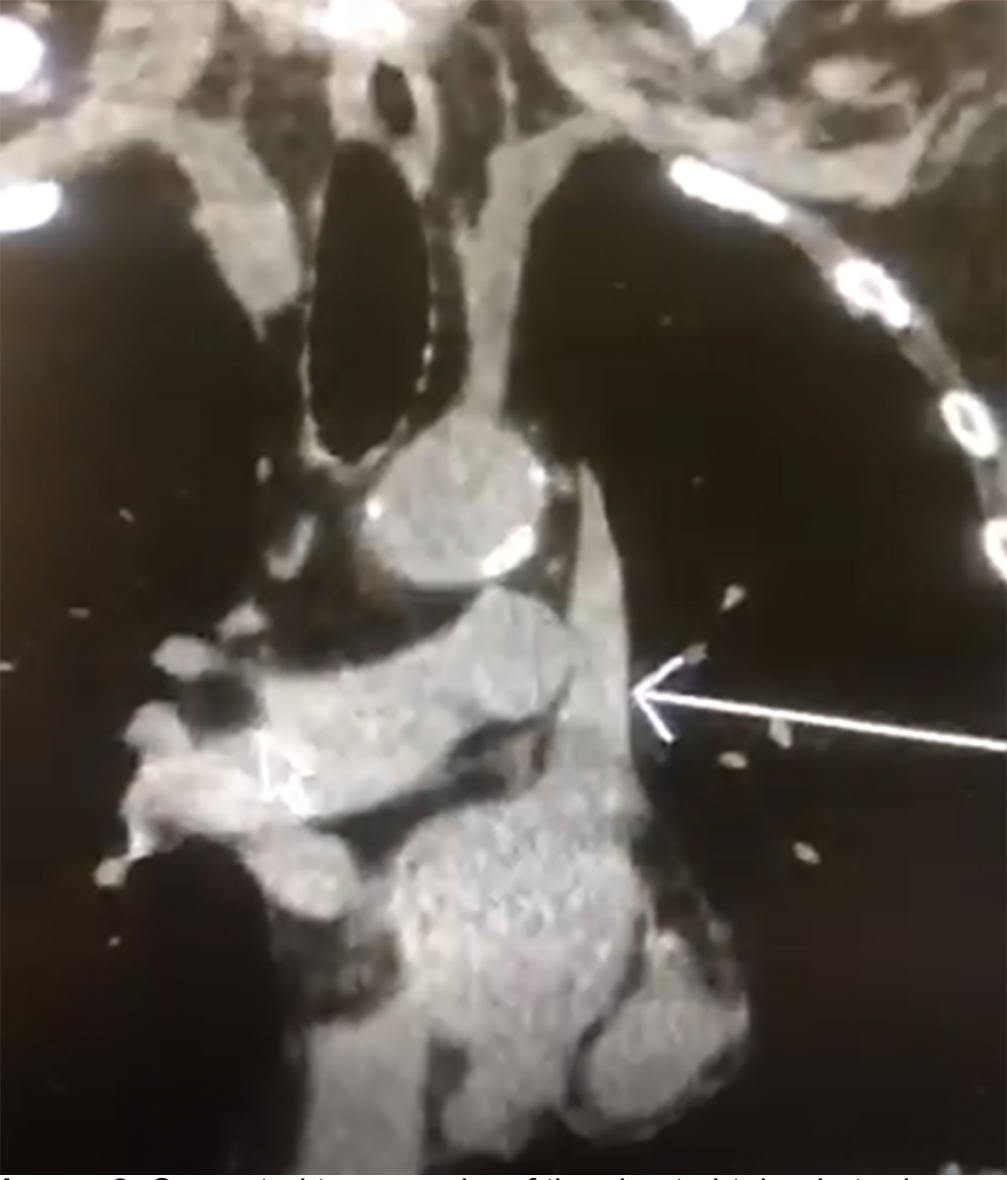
Computed tomography of the chest obtained at prior hospitalization demonstrates the presence of a persistent left-sided superior vena cava (arrow), as well as the complete absence of an accompanying right-sided superior vena cava.
